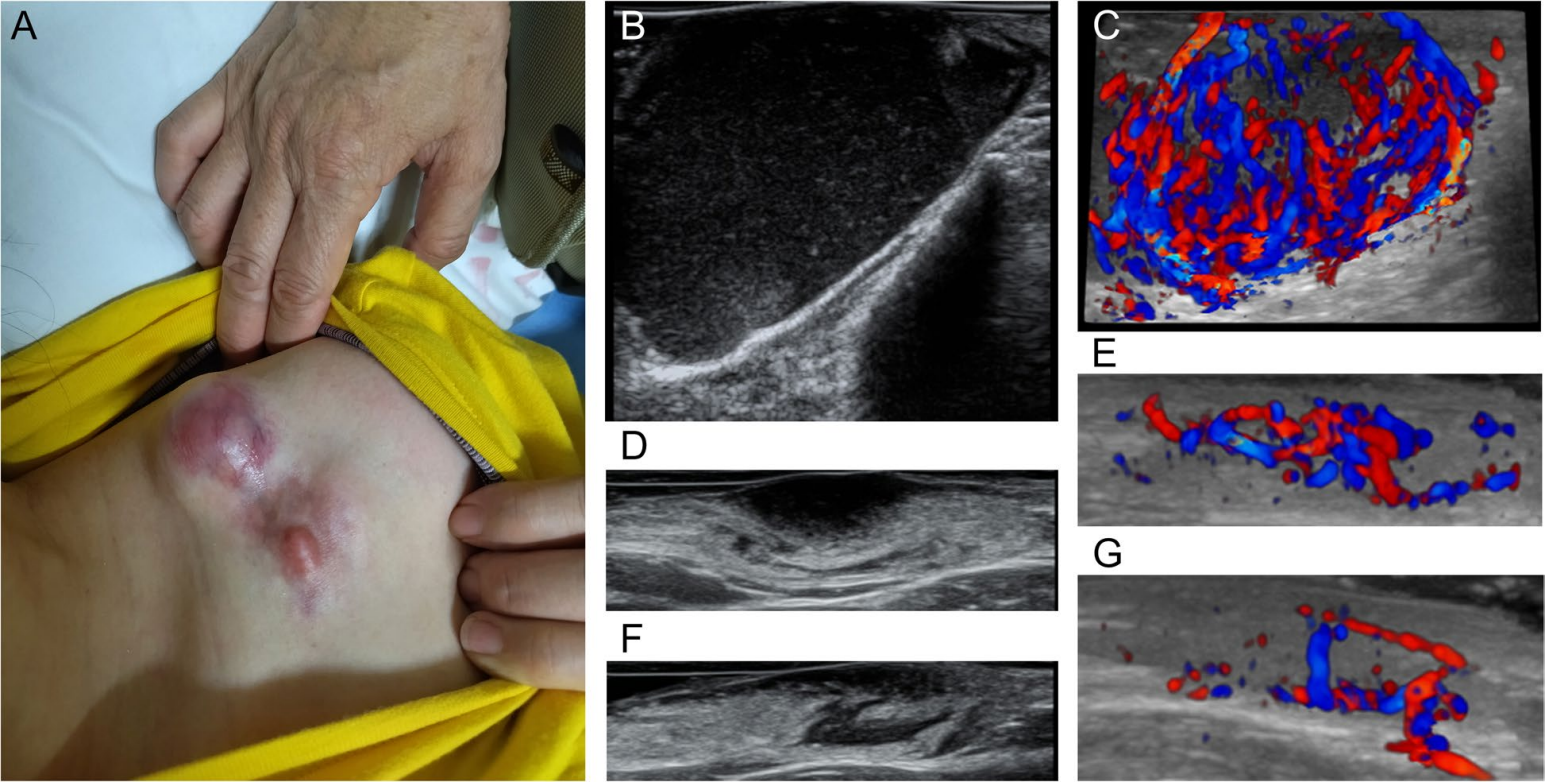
A DFSP with three nodules in the chest wall of a 22-year-old woman. B Transverse ultrasonogram (No. 1) revealing a well-defined, hypoechoic, homogeneous, and subcutaneous lesion. C 3-D color Doppler ultrasonogram revealing wrapped blood flow signal. D Transverse ultrasonogram (No. 2) revealing an ill-defined, hypoechoic with finger-like projections and posterior hyperechoic area, heterogeneous, and subcutaneous lesion. E 3-D color Doppler ultrasonogram revealing branch-shaped blood flow signal. F Transverse ultrasonogram (No. 3) revealing an ill-defined, mixed, heterogeneous, and subcutaneous lesion. G 3-D color Doppler ultrasonogram revealing branch-shaped blood flow signal
